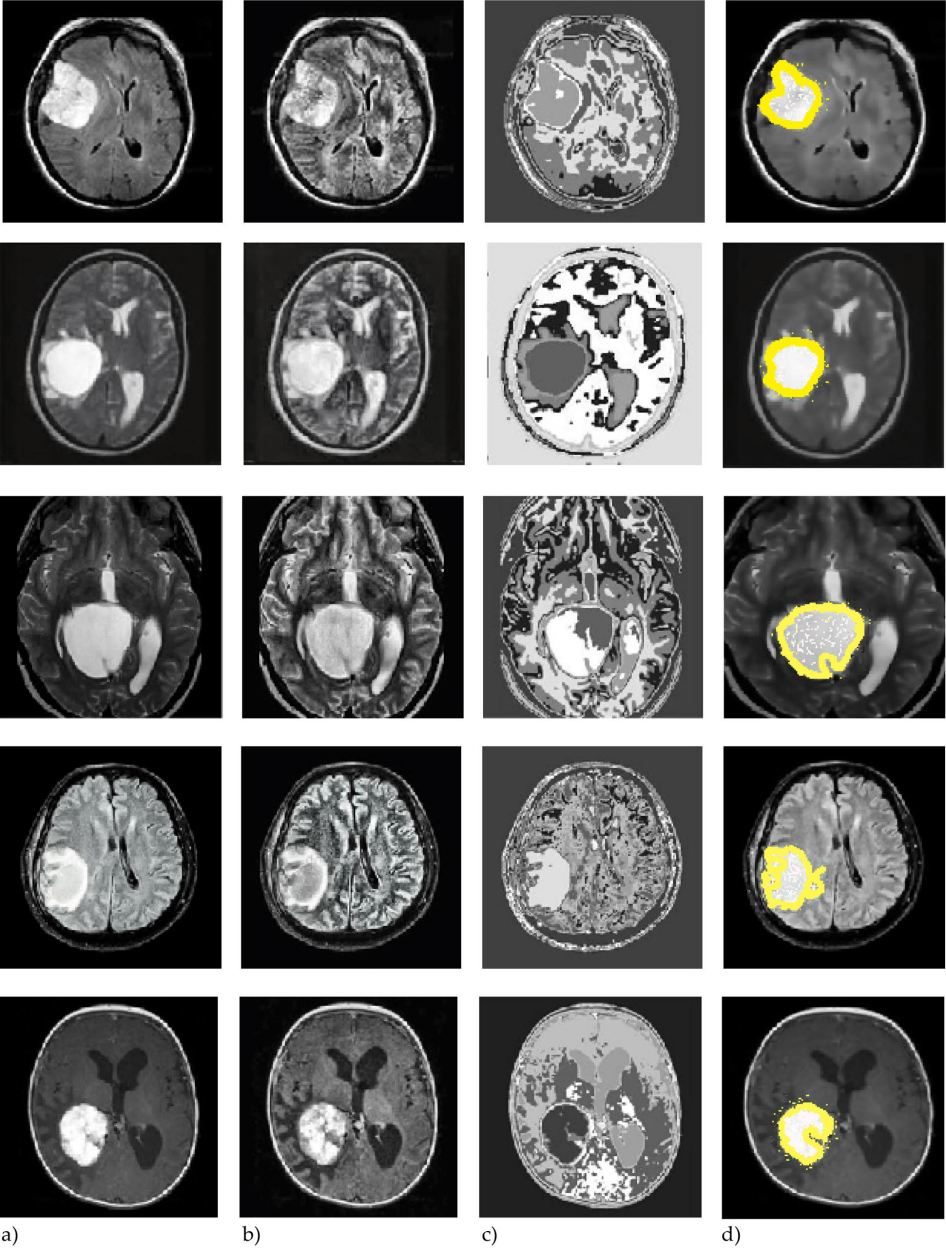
Accurate tumor extraction process from MRI images. (a) Tumor-containing primary MR images. (b) Enhanced pictures. (c) Fragments of tumor. (d) Segmented images.
